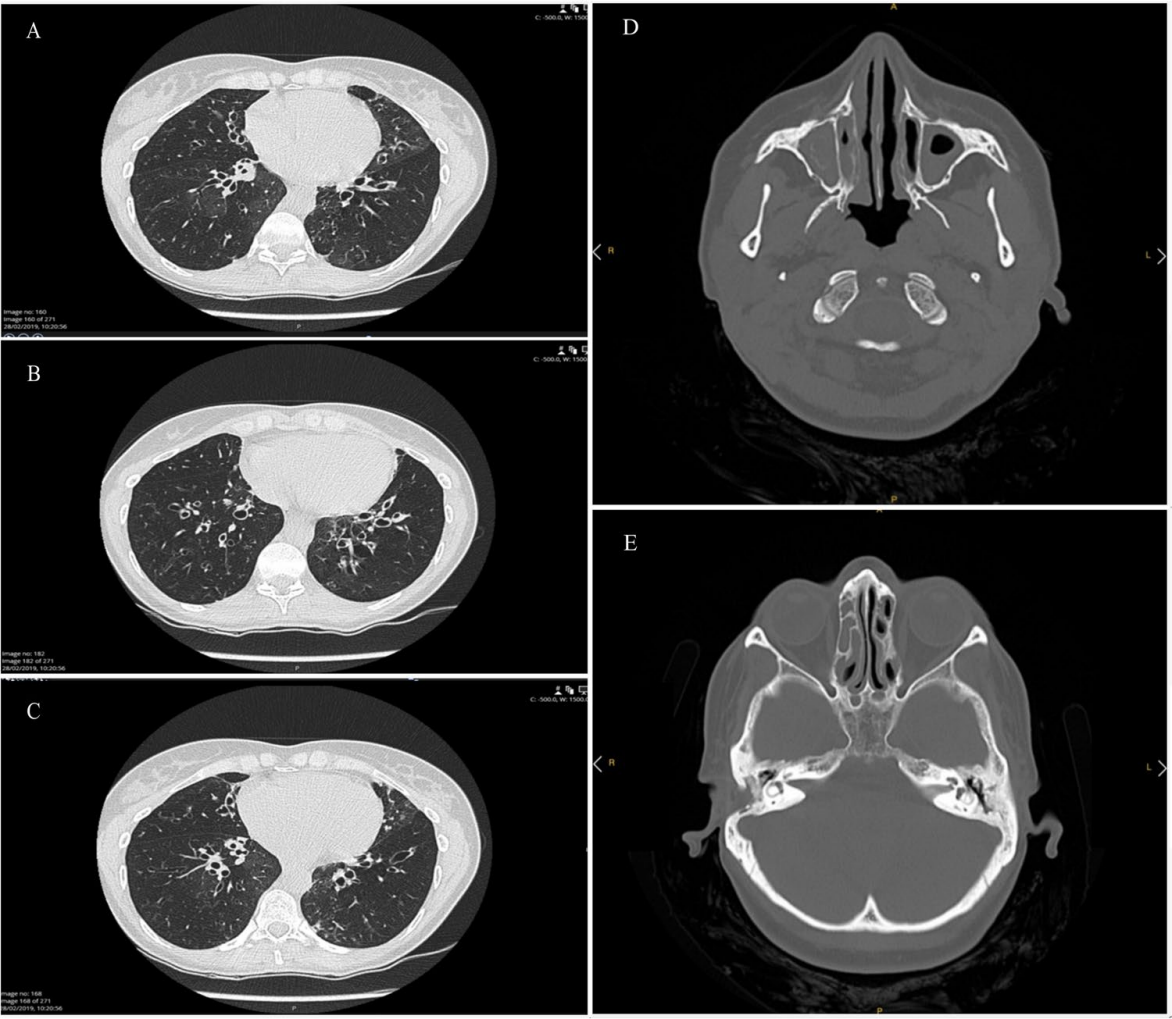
CT scan of the proband. A-C Thorax axial CT scan of the proband. A Note the presence of cystic bronchiectasis in the middle lobe and lower lobes. B and C. Note the presence of cystic bronchiectasis in the lower lobes. D Axial CT scan of paranasal sinuses showing obliteration of the maxillary sinus (complete in the right, partial in the left). E Axial CT scan shows opacification of the ethmoidal air cells bilaterally, more pronounced at the right. Right mastoidectomy
